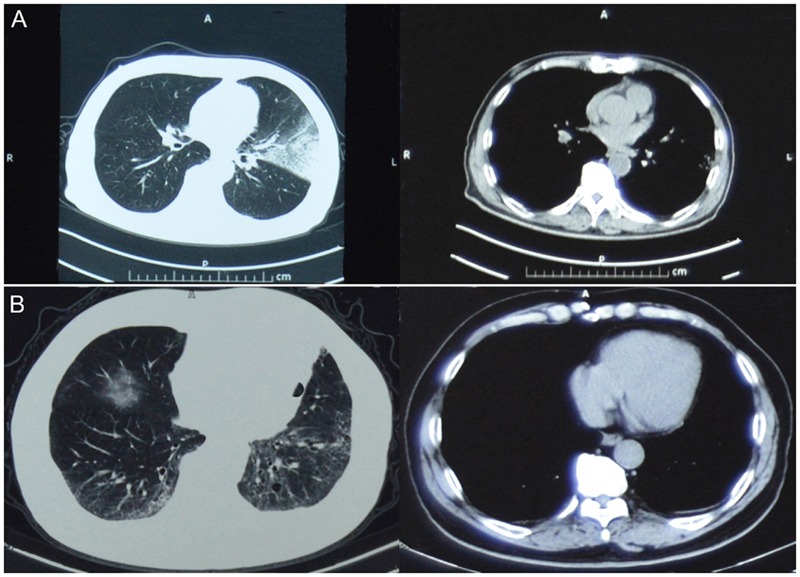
Computed tomography (CT) images of the patient. (A) and (B) show CT images of the patient infected with H7N9 virus on 25 April 2014 and of the patient infected with pH1N1 virus on 27 December 2015, respectively. Left and right CT images are lung and mediastinal window, respectively.
